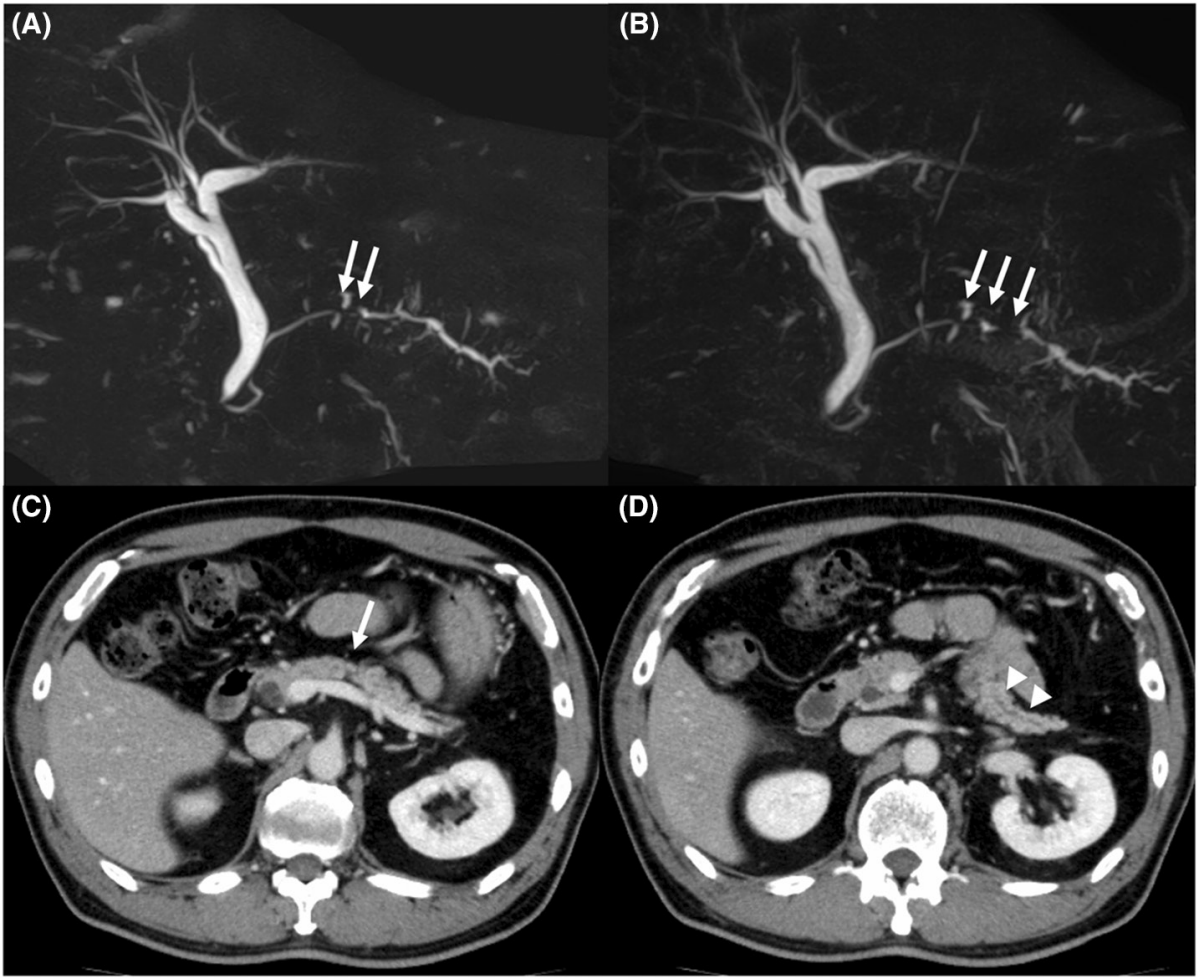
MRCP findings and CT findings. (A): MRCP showed stenosis of the MPD (arrow) in referral to our hospital. (B): MRCP showed that the stenosis of the MPD (arrow) became longer after 7 months. (C): CT showed that the pancreatic parenchyma of the body was atrophic (arrow). (D): CT showed that MPD of the tail was mildly dilated (arrow head).
